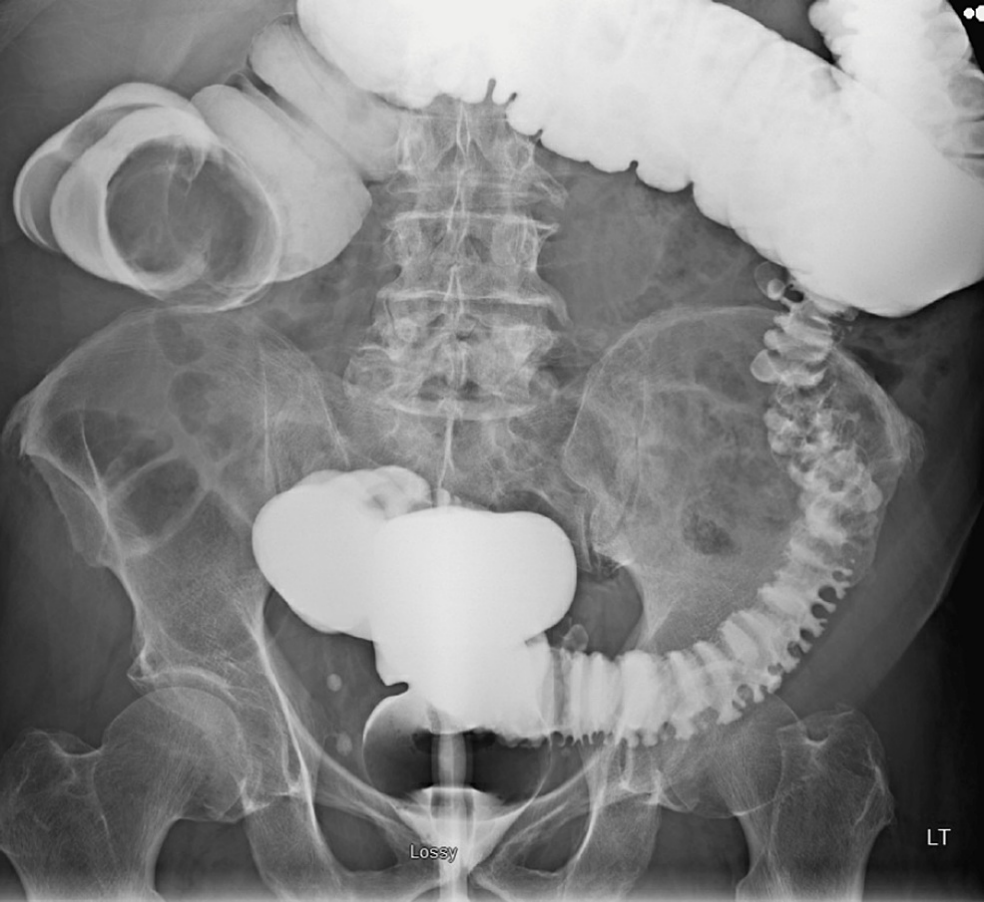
Barium enema followed by CT imaging revealed a round filling defect in the proximal transverse colon with complete obstruction. The location of the obstruction corresponded to a lipomatous lead point.
